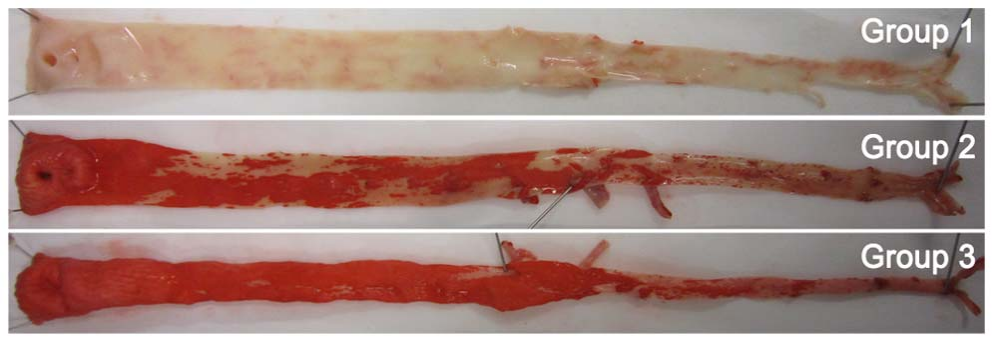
The vascular ultrasound of group 2 and group 3 in different weeks. Legend: (A) and (C): group 2 at week 12 and week 24; (B) and (D): group 3 at week 12 and week 24.
